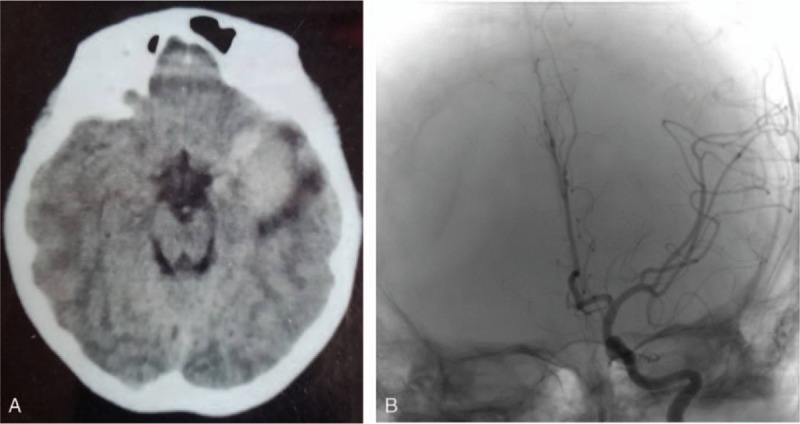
(A): CT scan revealing a high-density lesion in the left temporal lobe. (B): DSA showing negative findings.
